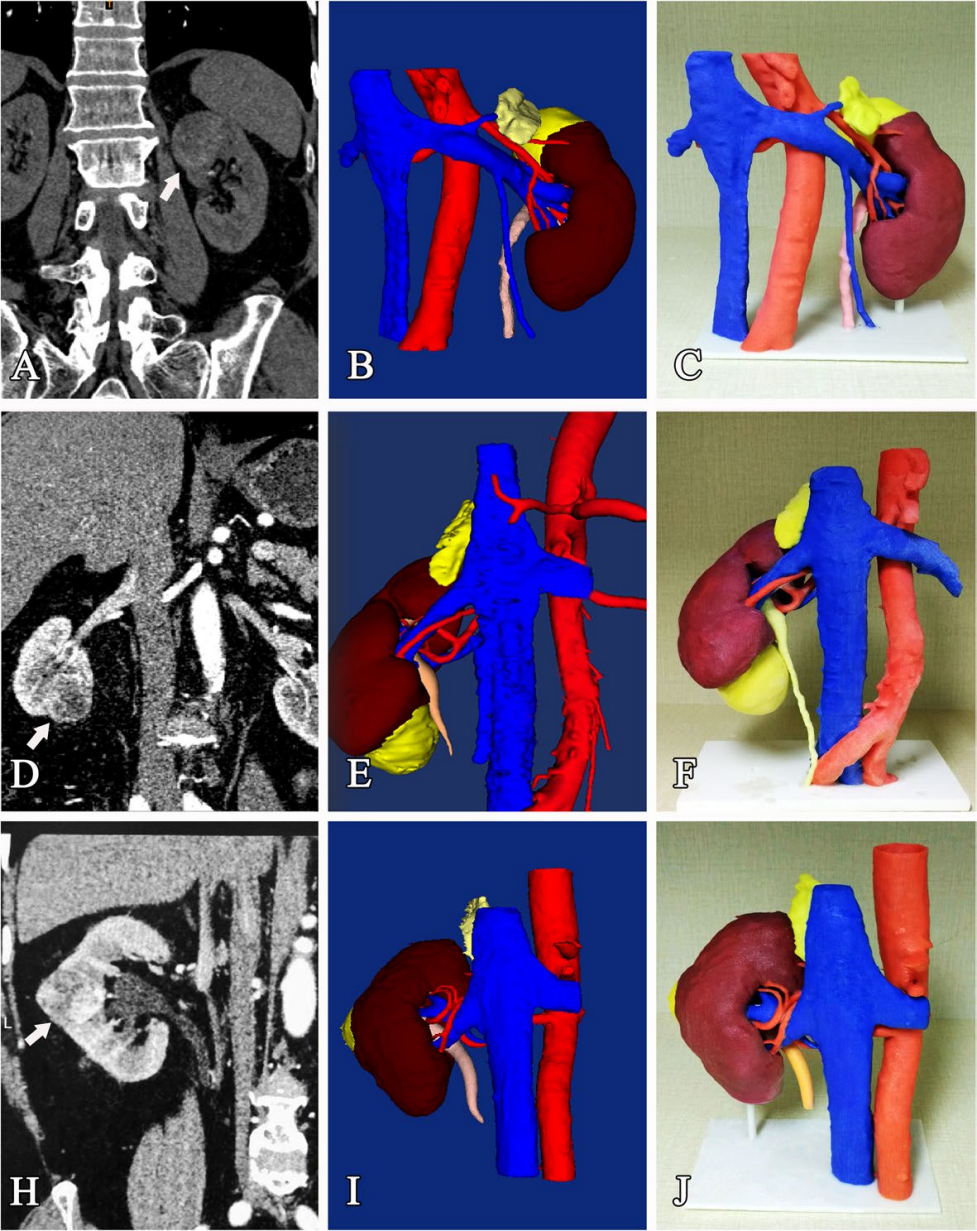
The CT-based 3D-IDMs and 3D-IPMs of the representative renal tumor cases (white arrow: the renal mass). A–C A 79-year-old male with a left-sided 4.8-cm renal mass on the upper pole (PUDUA score = 10P; R.E.N.A.L. nephrometry score = 8P). D–F A 53-year-old female with a right-sided 5.5-cm renal mass on the lower pole (PUDUA score = 11P; R.E.N.A.L. nephrometry score = 9P). H–J A 73-year-old male with a right-sided 4.0-cm renal mass in the mid part (PUDUA score = 10P; R.E.N.A.L. nephrometry score = 9P)
